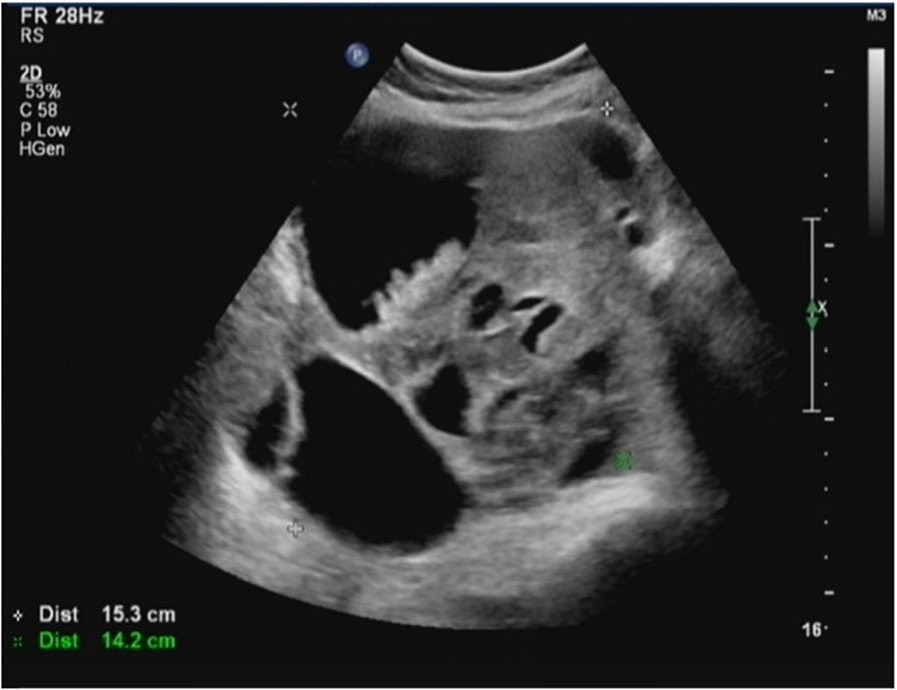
Ultrasound image at 20 weeks of gestation showed bulky masses behind uterus. The ultrasound image showed large cystic echoless mass behind uterus with obvious hyperechoic papillae in it
